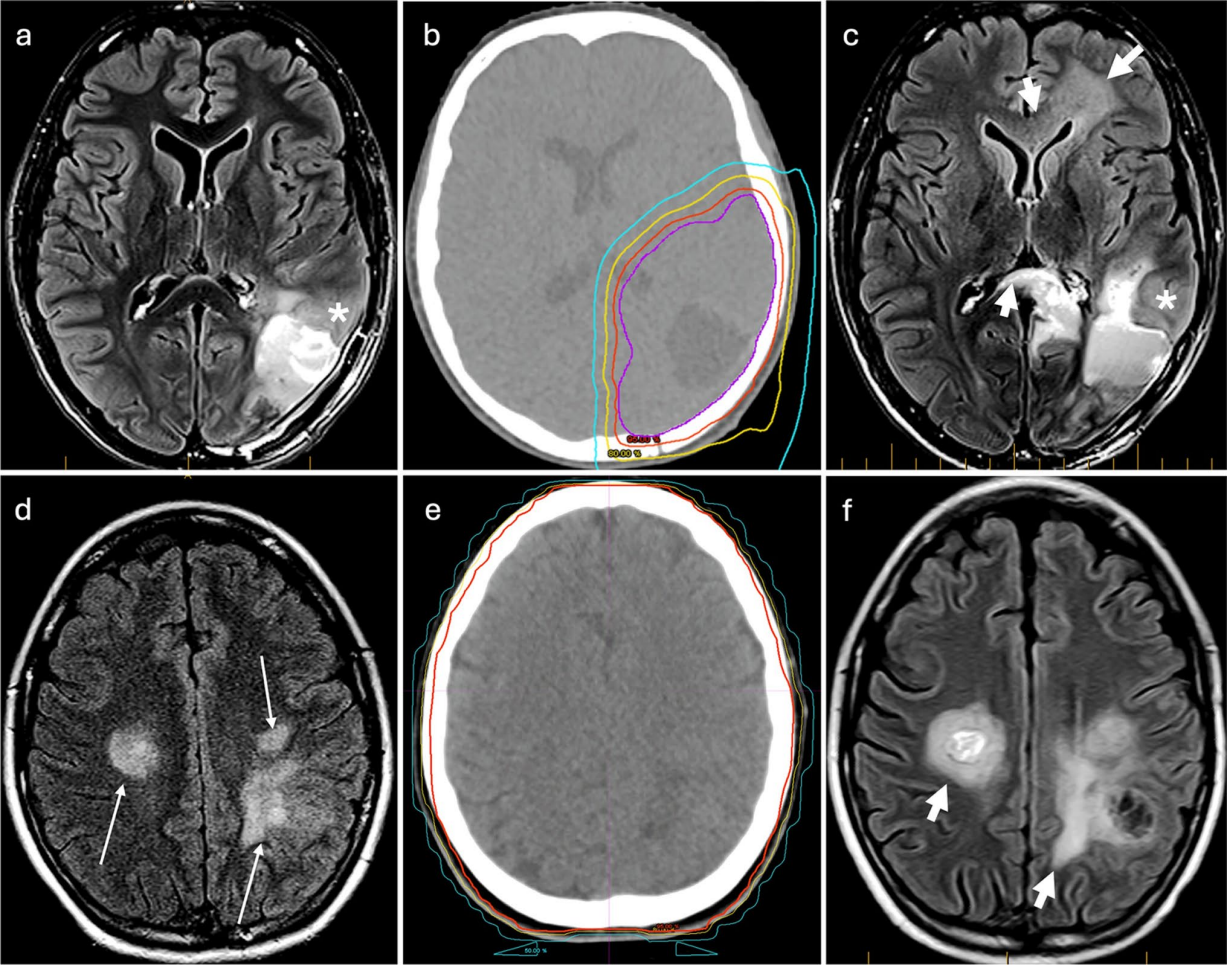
Patterns of recurrence in pediatric H3 G34-mutant DHG. (a–c, patient #11) Synchronous local and distal failure. a Axial T2-FLAIR image after partial resection demonstrates residual tumor anterior to the resection cavity in the right temporal lobe (asterisk). b The isodose lines of radiation planning are in the same plane as A. The patient received focal RT to a total dose of 59.4 Gy. The purple line shows the target volume; the red line shows the 95% dose line (56.4 Gy); the yellow line shows the 80% dose line (47.5 Gy); and the turquoise line shows the 50% dose line (29.7 Gy). The median splenium and genu of the corpus callosum and periventricular white matter around the left frontal horn are outside the 95% dose line. c Axial T2-FLAIR image on the same patient at recurrence at the same plane as in a and b demonstrates residual tumor anterior to the surgical resection cavity (*) and new ill-defined FLAIR abnormalities involving the splenium of the corpus callosum crossing the midline (thick arrow), within and adjacent to the 95% dose line, respectively, suggesting local failure. New ill-defined FLAIR abnormality in the genu of the corpus callosum and periventricular white matter around the left frontal horn (thick arrows), distal to the 95% dose line, suggesting distal failure. (d–f, patient #4) Local failure in a patient with gliomatosis cerebri developed after receiving 14.4 Gy focal radiation to the left parietal region. The patient was then transitioned to whole-brain radiation to 50.4 Gy (left parietal 64.8 Gy total). d Axial T2-FLAIR image demonstrates tumor in the left posterior frontal and parietal lobes as well as in the right posterior frontal lobe (thin arrows). e The isodose lines of radiation planning are in the same plane as d. The patient received whole-brain radiation to a total dose of 50.4 Gy. The red line shows the 95% dose line (47.9 Gy); the yellow line shows the 80% line (40.3 Gy); and the turquoise line shows the 50% line (25.2 Gy), all overlapping on the calvarium. The right frontal lobe received the same dose as the left frontal lobe. f Axial T2-FLAIR image of the same patient at the same plane as d and e at recurrence demonstrates further enlargement of the tumors in both the left posterior frontal and parietal lobes and the tumor within the right frontal lobe (thick arrows). As the patient received whole-brain radiation to a definitive dose, this progression was considered a local failure
